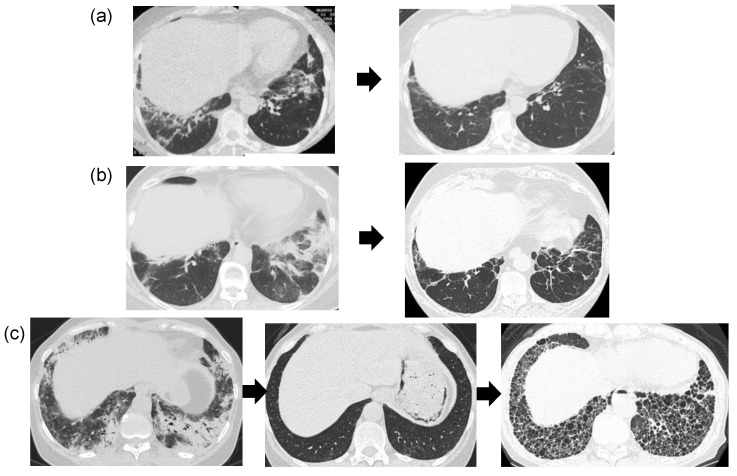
CT findings of anti-ARS antibody-positive ILD. (a) Prednisolone was administered, dense shadows along the bronchial vascular bundles improved, and prednisolone was discontinued after about 10 years. (b) Prednisolone and tacrolimus treatment left mild fibrosis after 4 years. (c) Prednisolone and cyclosporine A treatment achieved temporary improvement after 4 months, but fibrosis worsened after about 13 years.
